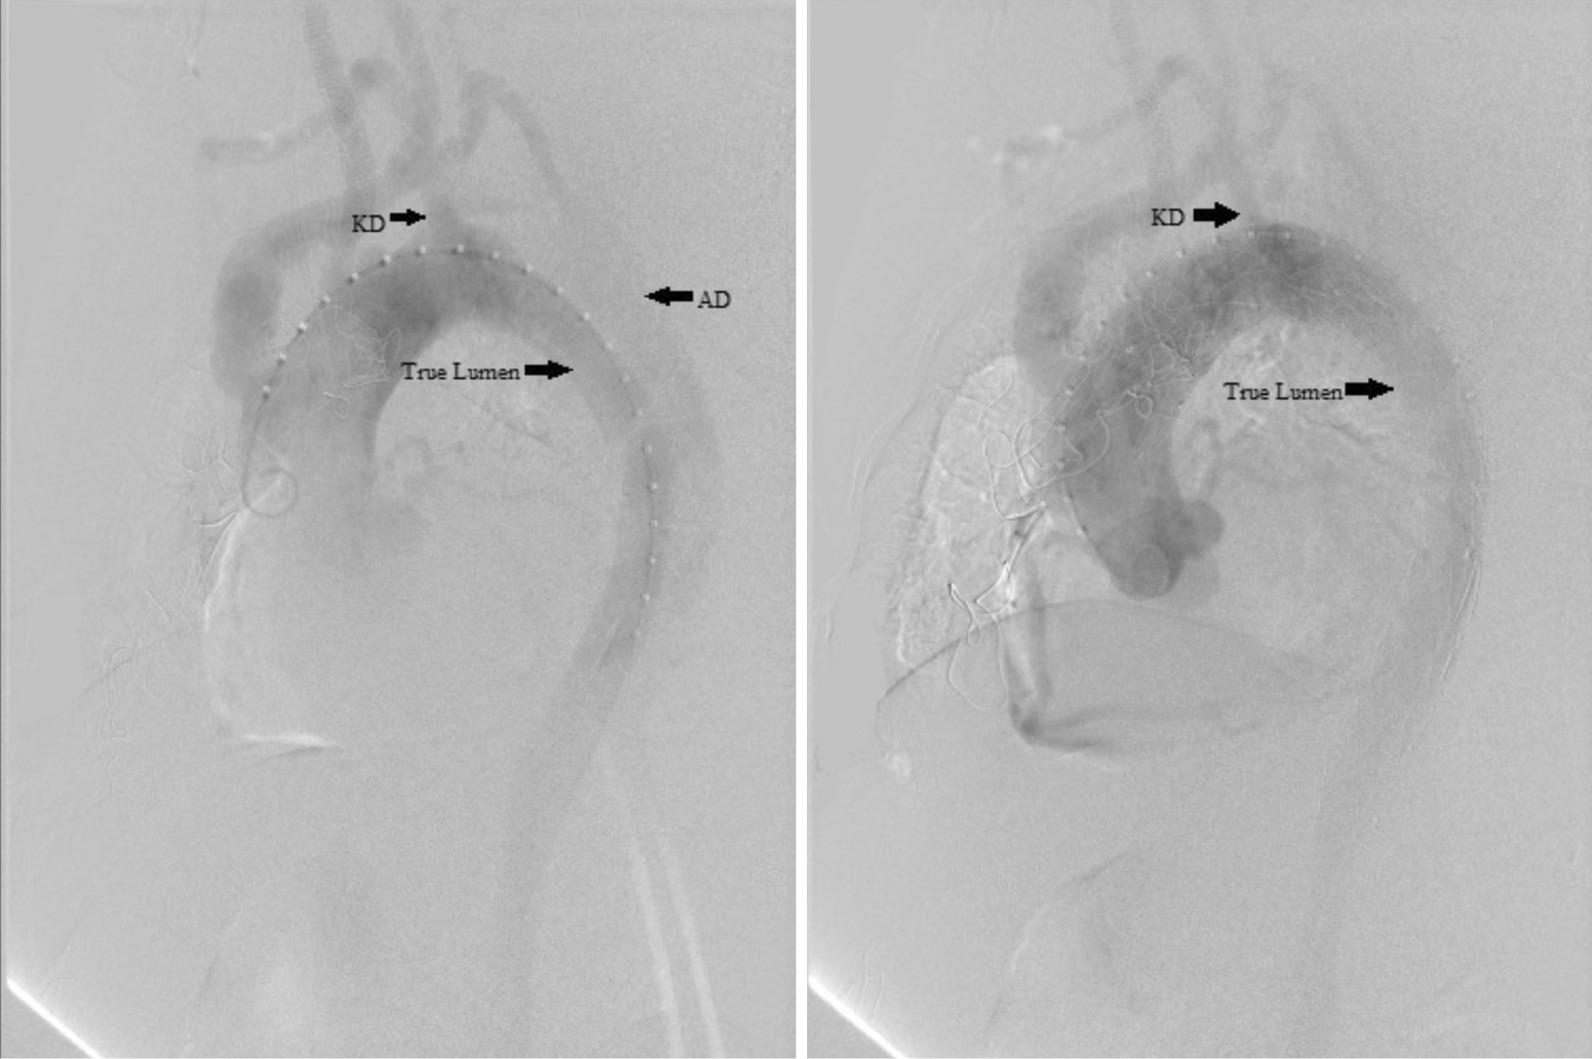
Radiography images during the operation
